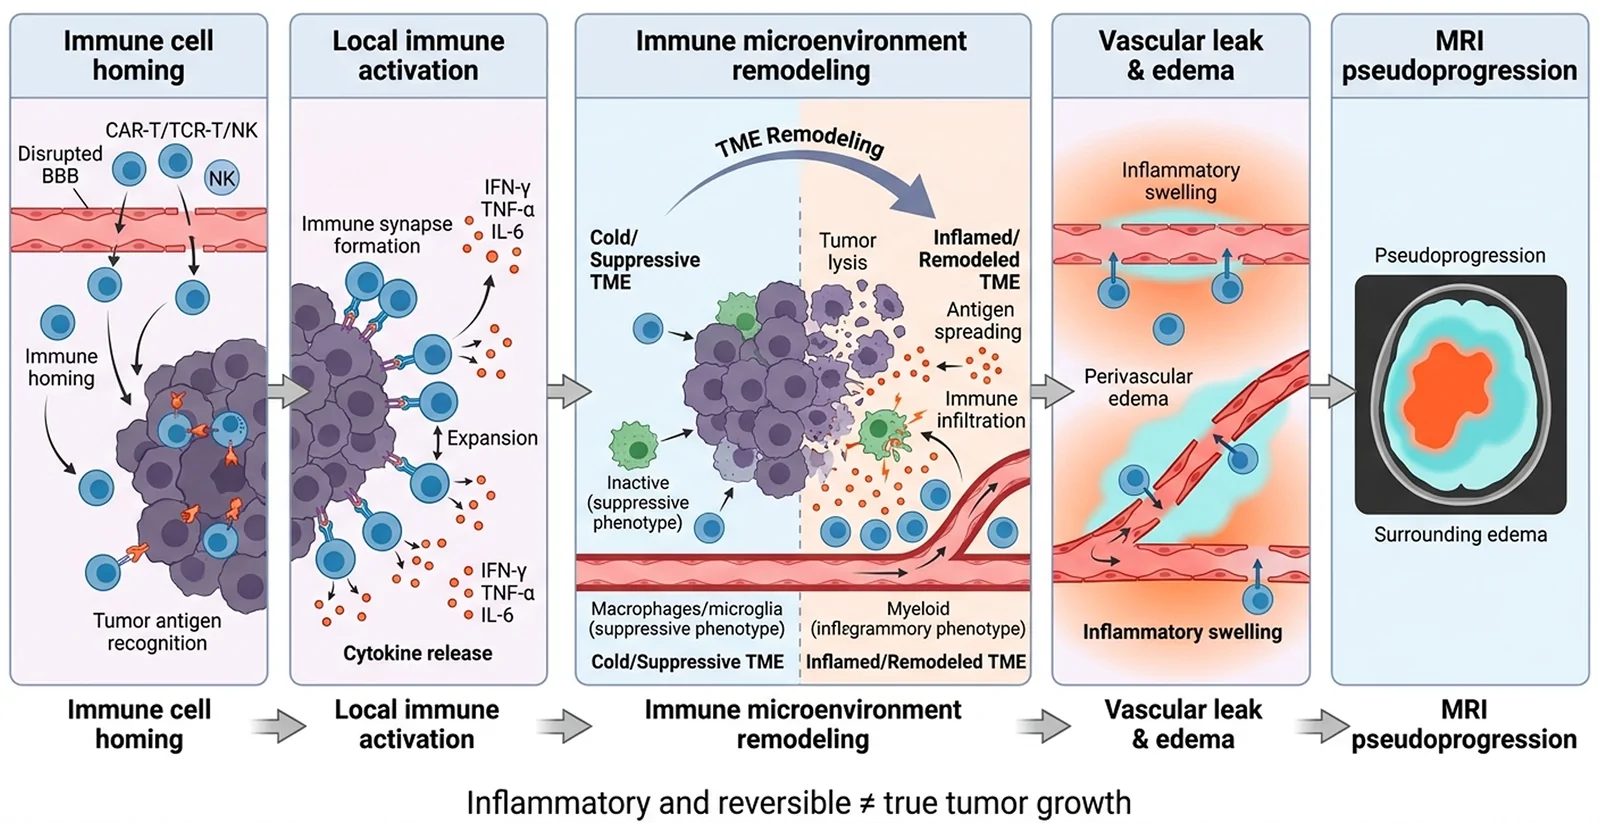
Stepwise TME remodelling cascade mediating cellular immunotherapy-associated PsP in glioblastoma (GBM): Infused CAR-T, TCR-T and NK cells cross damaged blood–brain barrier, home to tumours and bind glioma antigens. Immune synapse formation triggers lymphocyte expansion and robust secretion of IFN-γ, TNF-α and IL-6 to activate intratumoural inflammation. Tumour cell lysis releases neoantigens to induce antigen spreading, converting immune-suppressive cold TME into inflamed niche via three synergistic pathways: vascular normalization with increased permeability, pro-inflammatory polarization of tumour-associated myeloid cells, and disrupted tumour-myeloid symbiosis. Severe vascular leakage and peritumoural edema subsequently develop. These reversible inflammatory alterations manifest as transient enlarged contrast enhancement and widespread edema on gadolinium MRI, termed PsP instead of real tumour growth. Routine visual MRI evaluation fails to distinguish this inflammatory lesion from hypoxia-driven true tumour recurrence.
